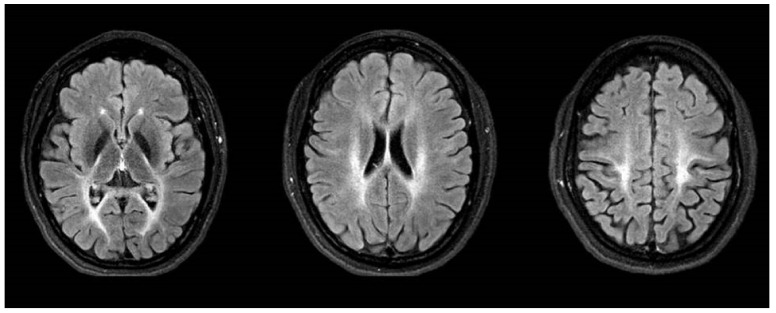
Cranial MRI in FLAIR sequence at the ages of 30 years showing severe supra- und infratentorial leukoencephalopathy with hypointense pallidum.
